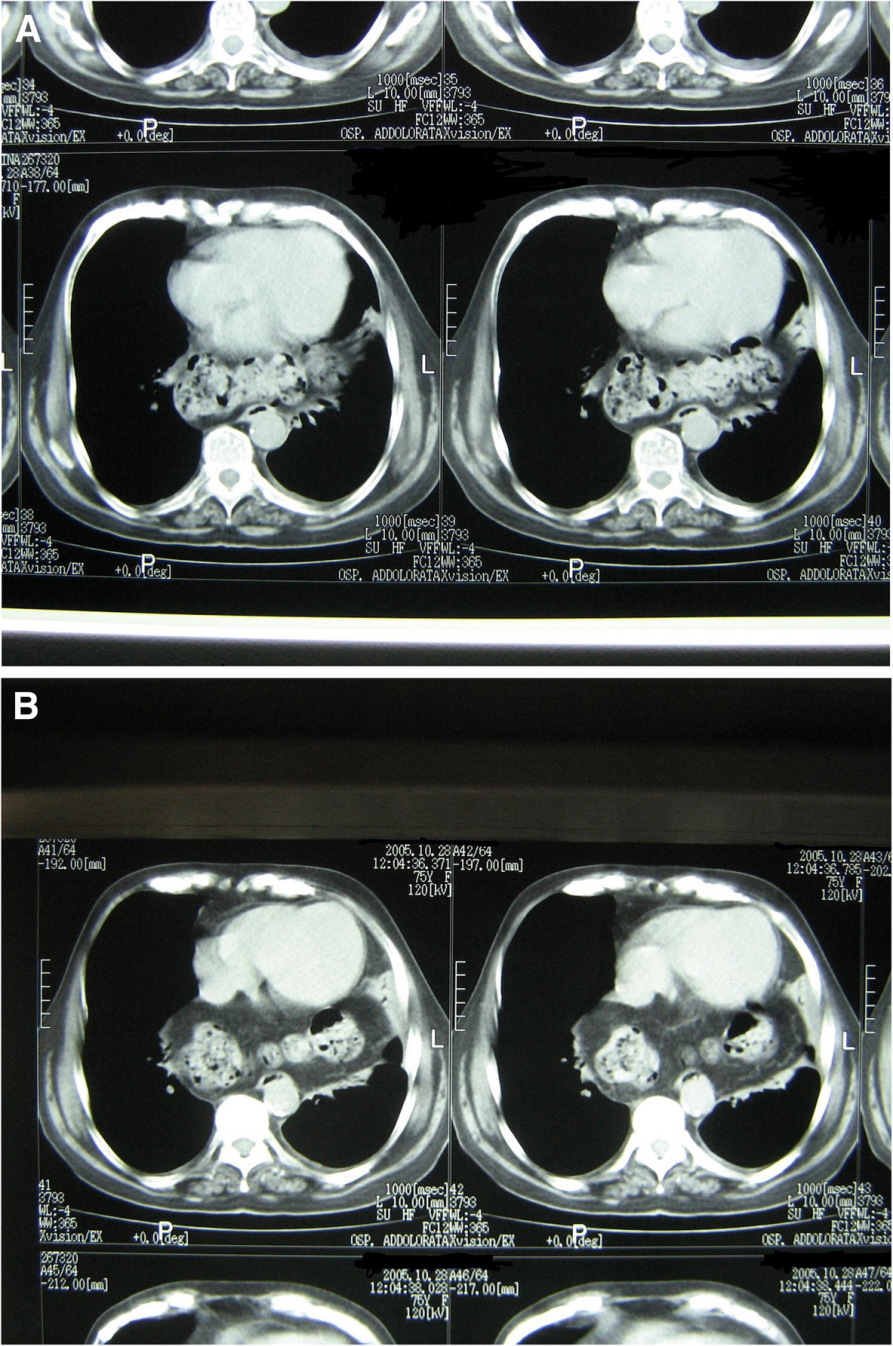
(A,B) Axial CT scan of the chest. Gas-filled large bowel loops are visible behind the heart, laying anteriorly to the spine and the aorta, with part of the abdominal fat.
